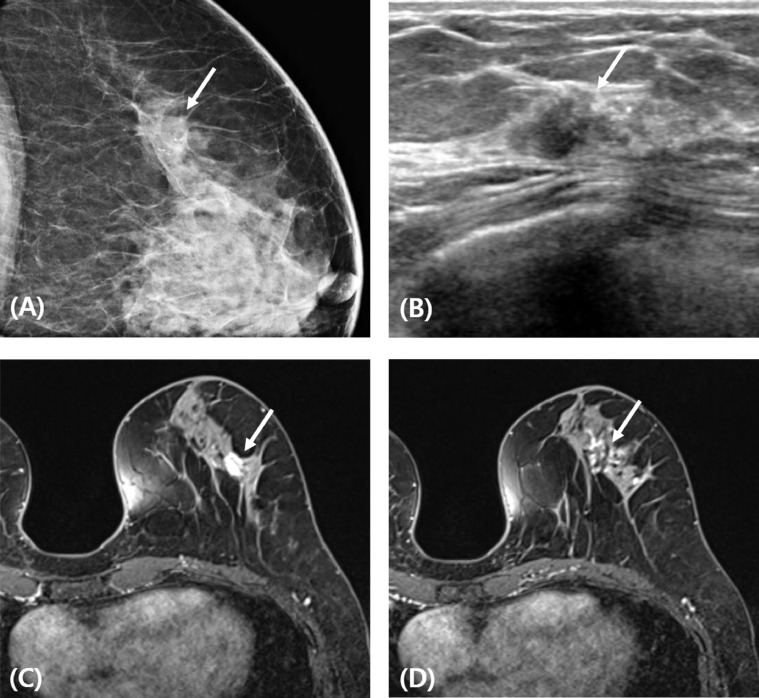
Preoperative imaging findings. (A) An irregularly shaped mass with intra- and extra tumoral microcalcifications (arrow) on mammogram. (B) An irregularly shaped mass and irregular ductal change with intraductal calcifications (arrow) on ultrasound. (C,D) MR images demonstrate an approximately 1.3-cm irregularly shaped mass and focal non-mass enhancement (arrows) with a total extent of approximately 3.5 cm.
